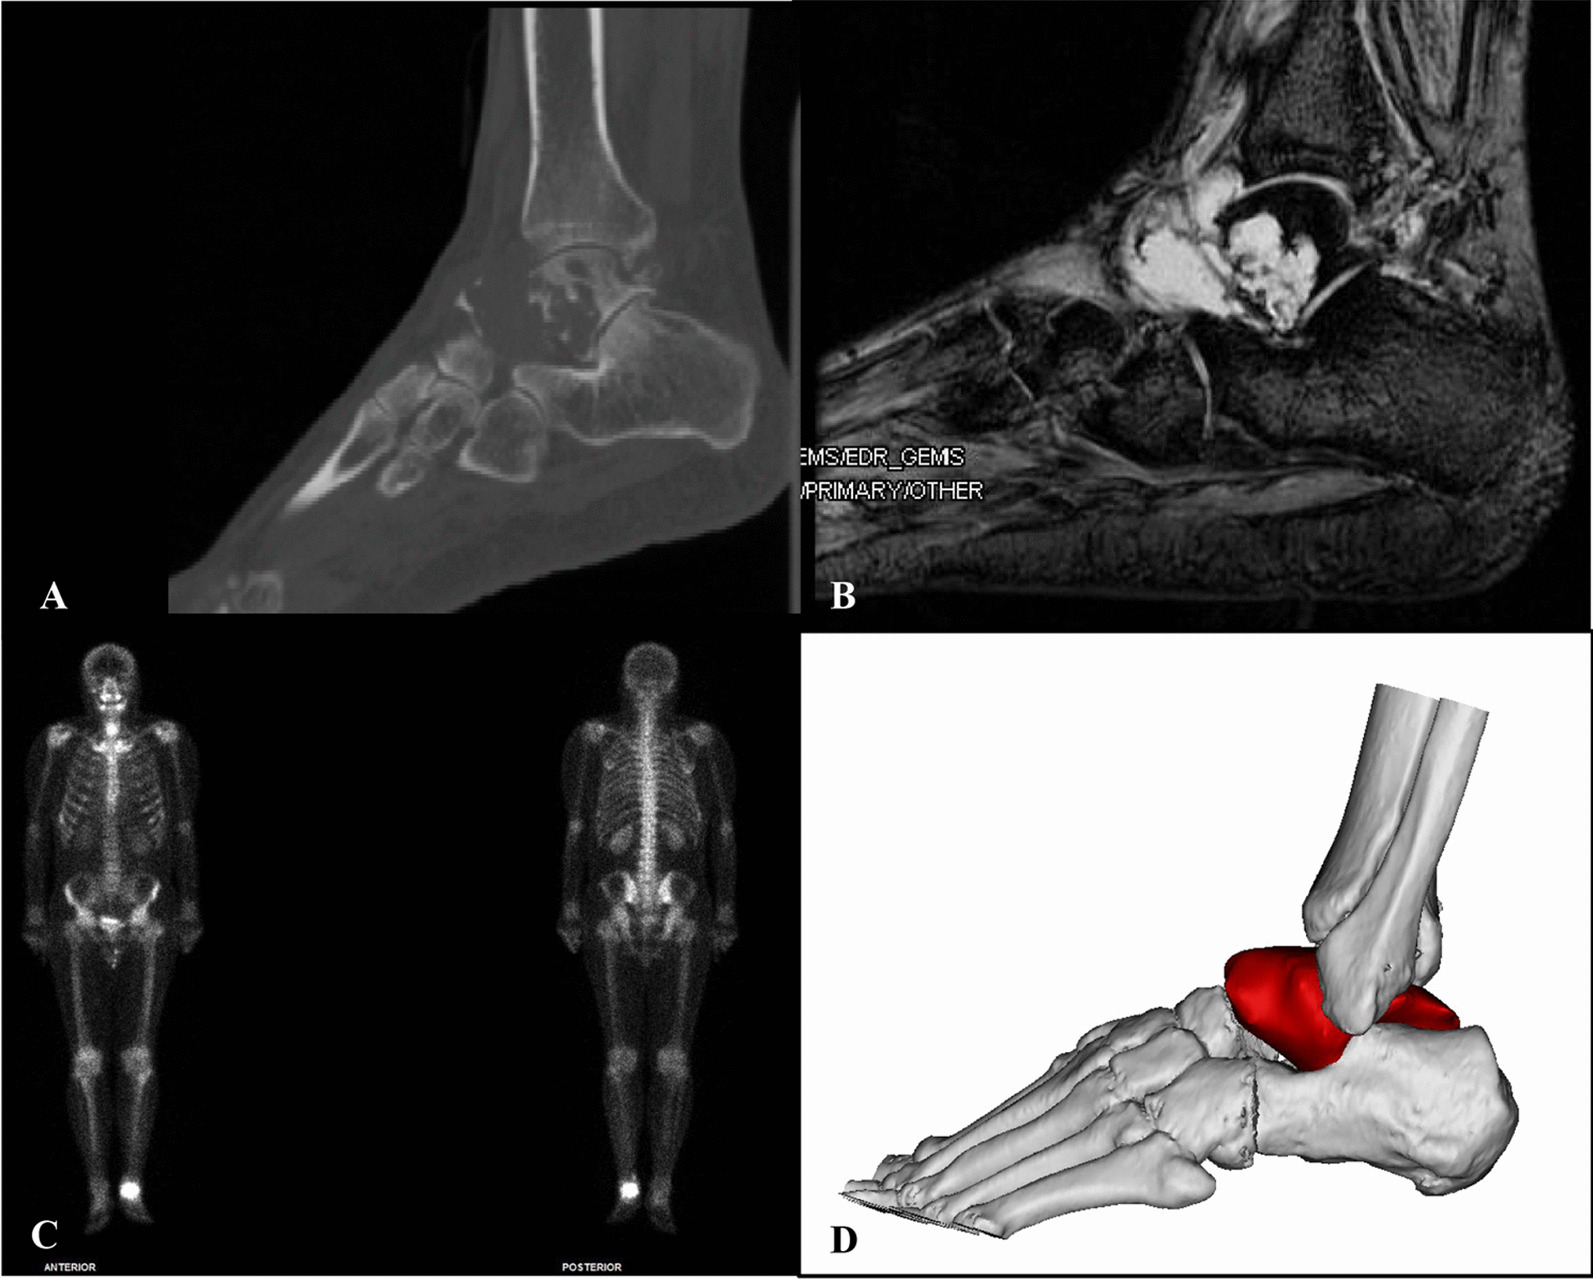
Preoperative imaging including CT (A), MRI (B), and SPECT (C) tumor model (D) of the patients
